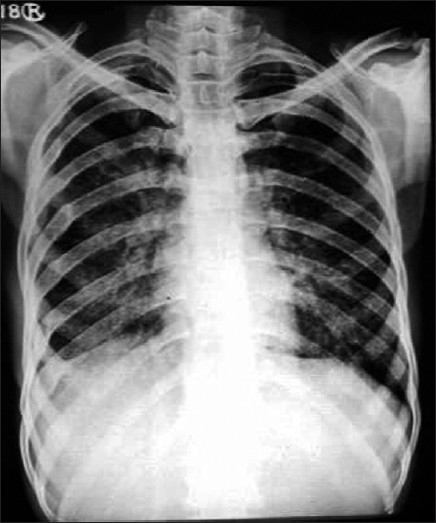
Chest radiograph showing bilateral mid and lower zone miliary nodular opacities
